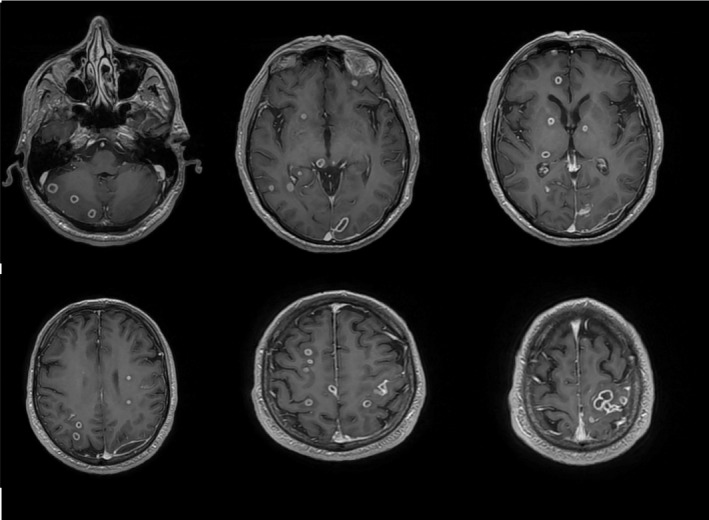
MRI Brain series revealing innumerable septic emboli to brain parenchyma
